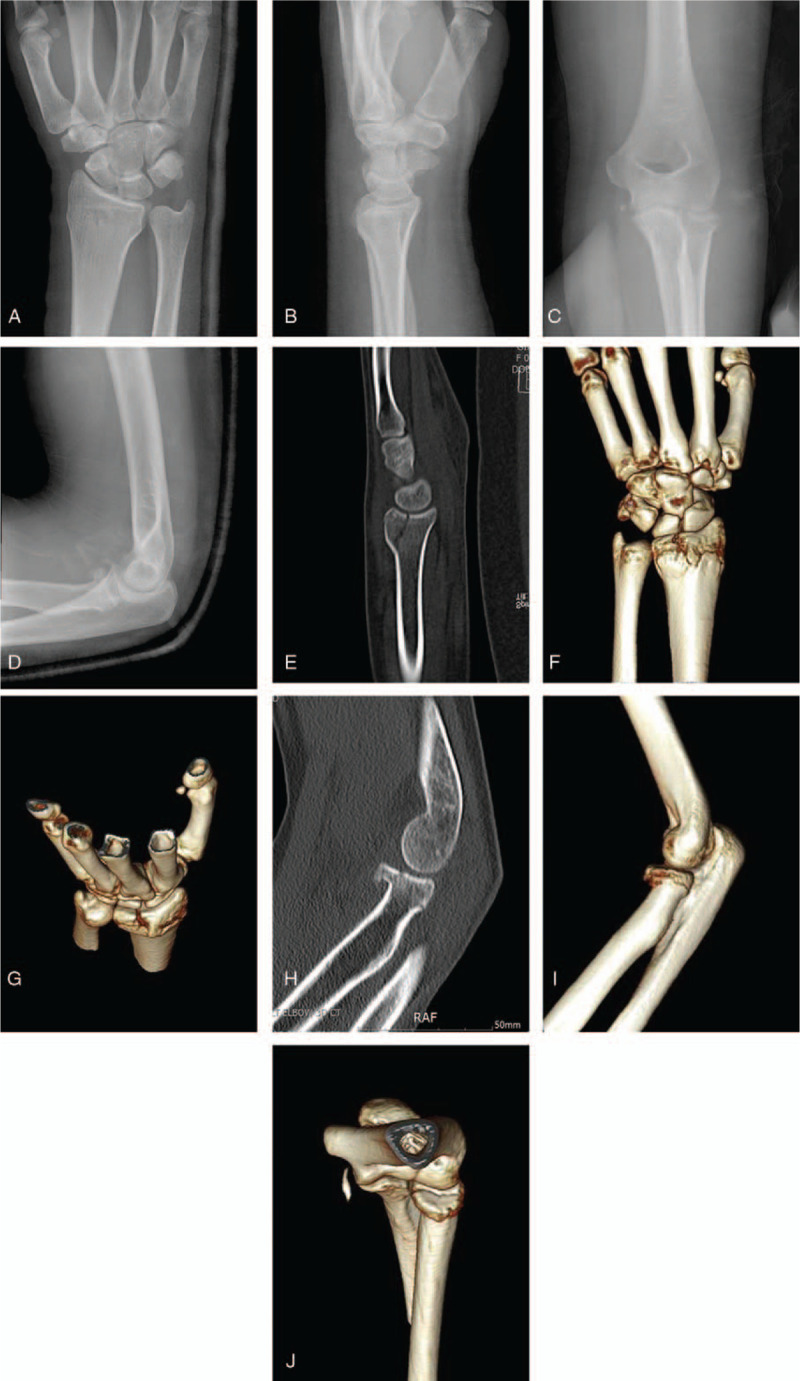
Preoperative plain radiographs of case 2. (A, B) Anteroposterior and lateral X-ray images of the wrist (A, B) and the elbow (C, D) and CT images of the wrist (E-G) and the elbow (H-J) show an AO B2 undisplaced distal radius fracture and Mason type III radial head fracture.
